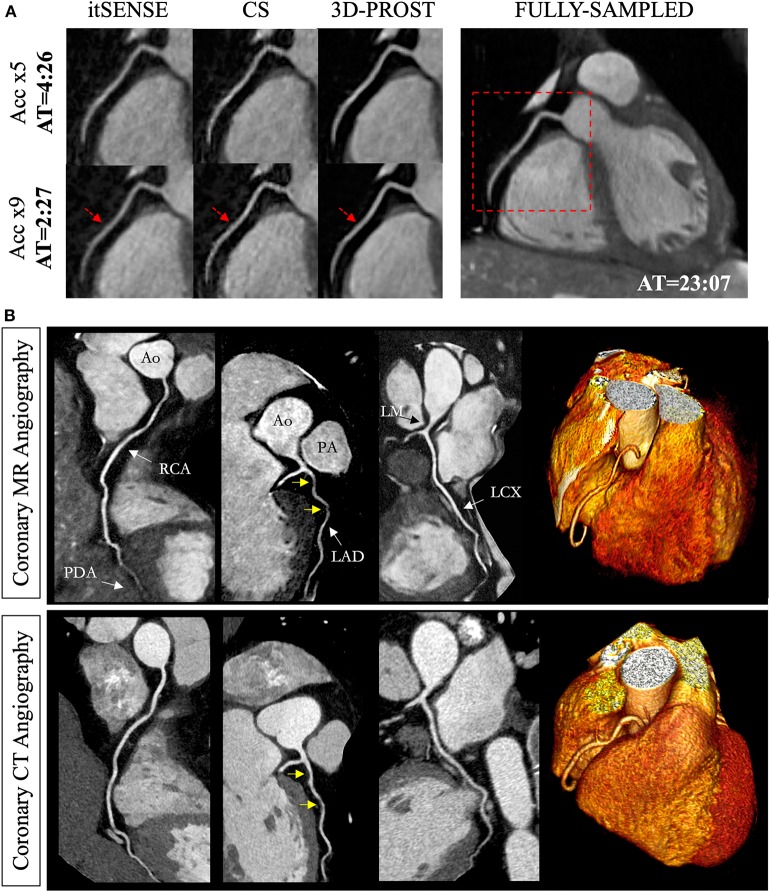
Reconstruction comparisons for coronary MR angiography. (A) Example of reformatted images of the right coronary artery from three healthy subjects acquired at 1.2 mm^3^ isotropic resolution with a fully sampled whole-heart coronary MR angiography sequence, and with 2 undersampled acquisitions (5- and 9-fold acceleration with variable density sampling), reconstructed using iterative SENSE (itSENSE), wavelet-based compressed sensing reconstruction (CS) and a 3D patch-based approach [3D-PROST (56)]. 3D-PROST provides higher image quality and sharpness (red arrows) than itSENSE and CS for both acceleration factors, achieving similar image quality to the fully sampled reference. Acquisition times (AT) are expressed as min:s. (B) Non-contrast whole-heart sub-millimeter isotropic CMRA images of 53-year-old female patient acquired in 10 min 7 s (5-fold undersampling) and reconstructed with 3D-PROST (56) and non-rigid motion correction (57). Visual comparison with contrast-enhanced Computed Tomography angiography (bottom row) shows good agreement and delineation of the coronary arteries with the free-breathing 3D patch-based motion corrected CMRA framework.
